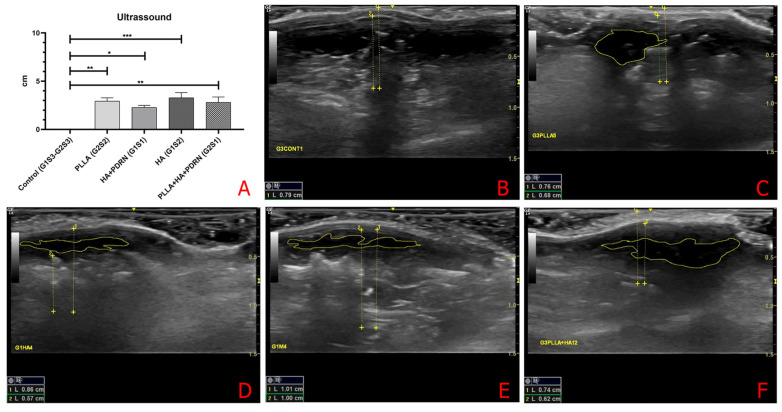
Ultrasound-Based Assessment of Dermal Hypoechogenicity. High-frequency ultrasound evaluation of dermal hypoechogenicity after treatment. Images were acquired using a Logiq E R7 system (GE Healthcare, Chicago, IL, USA). Quantitative measurements were performed using ImageJ^®^ software (version 1.54g, National Institutes of Health, Bethesda, MD, USA), and statistical analysis was conducted using GraphPad Prism version 9.0 (GraphPad Software, San Diego, CA, USA). (A) Quantitative analysis of dermal hypoechogenicity showing significant differences among treated groups and the untreated control. (B) Untreated control skin (G1S3/G2S3). (C) PLLA-treated skin (G2S2). (D) HA and PDRN-treated skin (G1S1). (E) HA-treated skin (G1S2). (F) Skin treated with the combined PLLA, HA, and PDRN formulation (G2S1). Asterisks indicate statistically significant differences (* *p* < 0.05;  *p* < 0.01; * *p* < 0.001), according to the comparisons shown in panel A.
